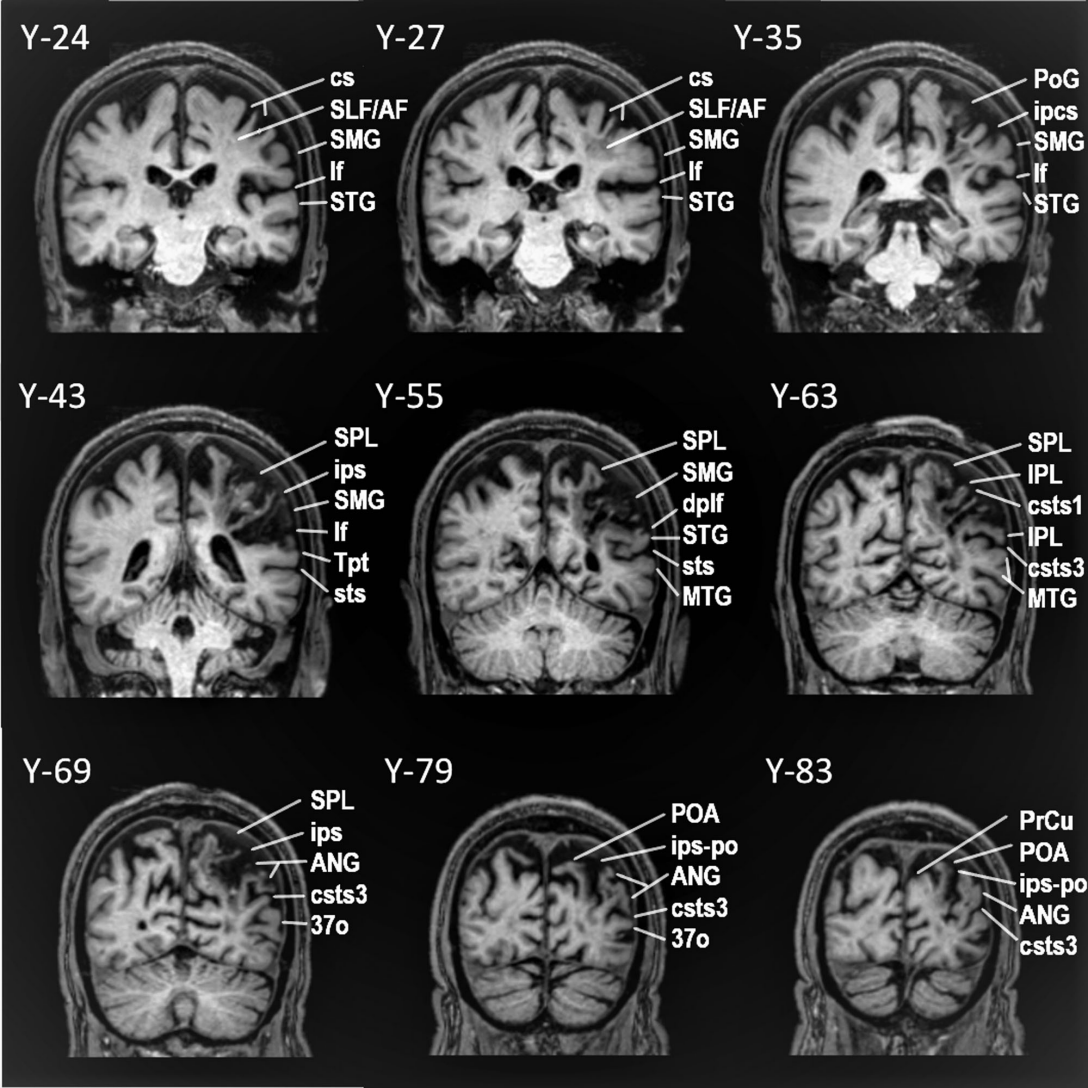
Successive coronal MRI images in MNI stereotaxic coordinates (Y) depicting the lesion of patient TA with damage to the dorsal posterior language region. The lesion starts posterior to the central sulcus (cs) and after Y-24. At Y-27, the white matter below SMG and postcentral gyrus areas 2, 1, 3b is damaged affecting SLF III fibers (originating from the SMG), SLF II fibers (originating from ANG), and AF fibers (originating from Tpt. At Y 31, a small area of anterior SMG is added to the white matter lesion. At Y-35 and Y-39, the lesion includes SMG and the white matter below. At Y-43, the whole SMG, the white matter below and a small part of area Tpt, that is the caudal STG, is damaged, leaving primary acoustic areas on Heschl’s gyrus intact. Further posteriorly, at Y-47, -51, -55 as well as -59, damage continues to include the whole SMG and SPL (Superior Parietal Lobe), occupying a larger part of STG (see Y-55). At Y-63, superior and inferior parietal lobule areas, including areas PFm and PG, as well as the white matter below, until csts3 are affected. At Y-67 till Y-71, the superior and inferior parietal lobule areas, including ANG, as well as the underlying white matter until the area just below csts2, are lesioned (see Y-69). At Y-75, Y-79, and Y-83, the precuneus, superior and inferior parietal lobule areas until csts3 are affected, while at Y-83, the lesion includes the areas of POA and the whole ANG until csts3. The lesion continues further posteriorly to parieto-occipital areas, affecting even occipital areas such as area 19, in Y-98, where the lesion ends. Brain areas are topologically defined according to the atlas of the morphology of the human cerebral cortex in the MNI Stereotaxic Space^67^. 3a: area 3a; 37o: lateral occipitoparietal area 37; AF Arcuate Fasciculus; ANG angular gyrus; cs central sulcus; csts1, csts3 caudal superior temporal sulcus, 1st and 3rd ramus; IPL inferior parietal lobule; ipcs inferior postcentral sulcus; ips intraparietal sulcus; ips-po intraparietal sulcus, paroccipital part; lf lateral fissure; MTG middle temporal gyrus; POA parieto-occipital arcus; PoG postcentral gyrus; PrCu precuneus; SLFII/III superior longitudinal fasciculus, subdivision II/III; SMG supramarginal gyrus; SPL superior parietal lobule; STG superior temporal gyrus; sts superior temporal sulcus; Tpt caudal superior temporal cortical region.
